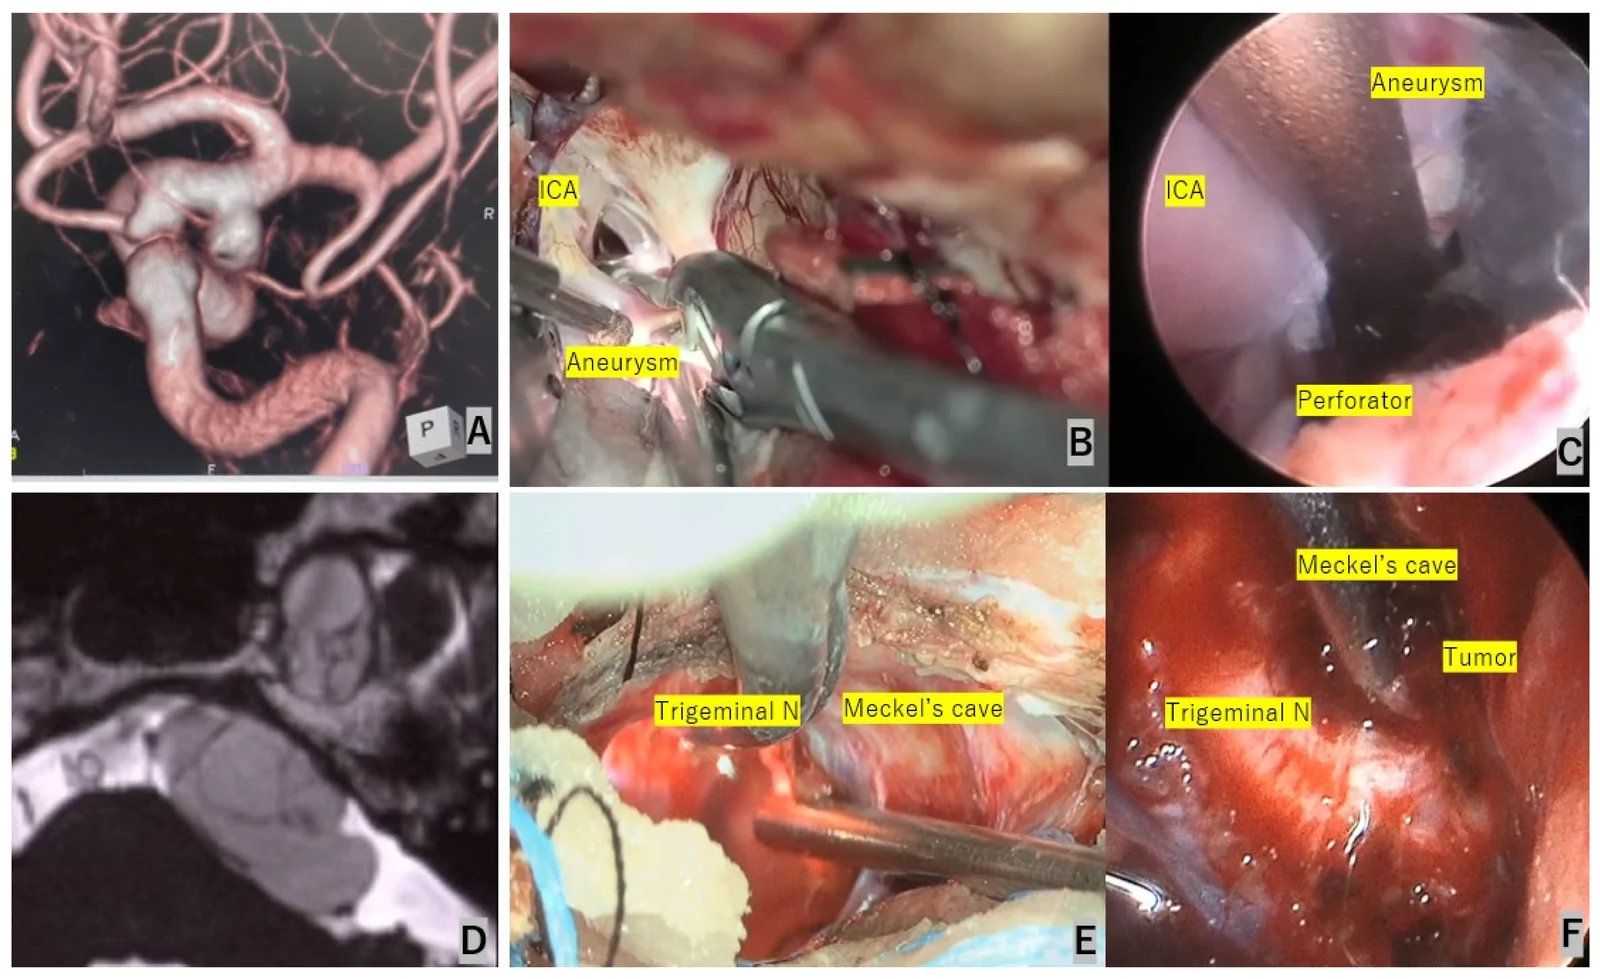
Representative applications of neuroendoscopic assistance during aneurysm and Meckel’s cave surgery. (A–C) Clip and perforator confirmation during clipping of an intracranial aneurysm. (A) Preoperative three-dimensional computed tomography angiography demonstrating the aneurysm. (B) Exoscopic view during aneurysm clipping. (C) Endoscopic view providing additional visualization of the aneurysm neck and perforating arteries hidden from the direct exoscopic line of sight. (D–F) Visualization of Meckel’s cave during resection of a trigeminal schwannoma. (D) Preoperative T2WI magnetic resonance imaging showing the tumor extending into Meckel’s cave. (E) Exoscopic intraoperative view. (F) Endoscopic view allowing inspection of Meckel’s cave and visualization of tumor portions hidden from the direct exoscopic line of sight. ICA, internal carotid artery; Trigeminal N, trigeminal nerve.
